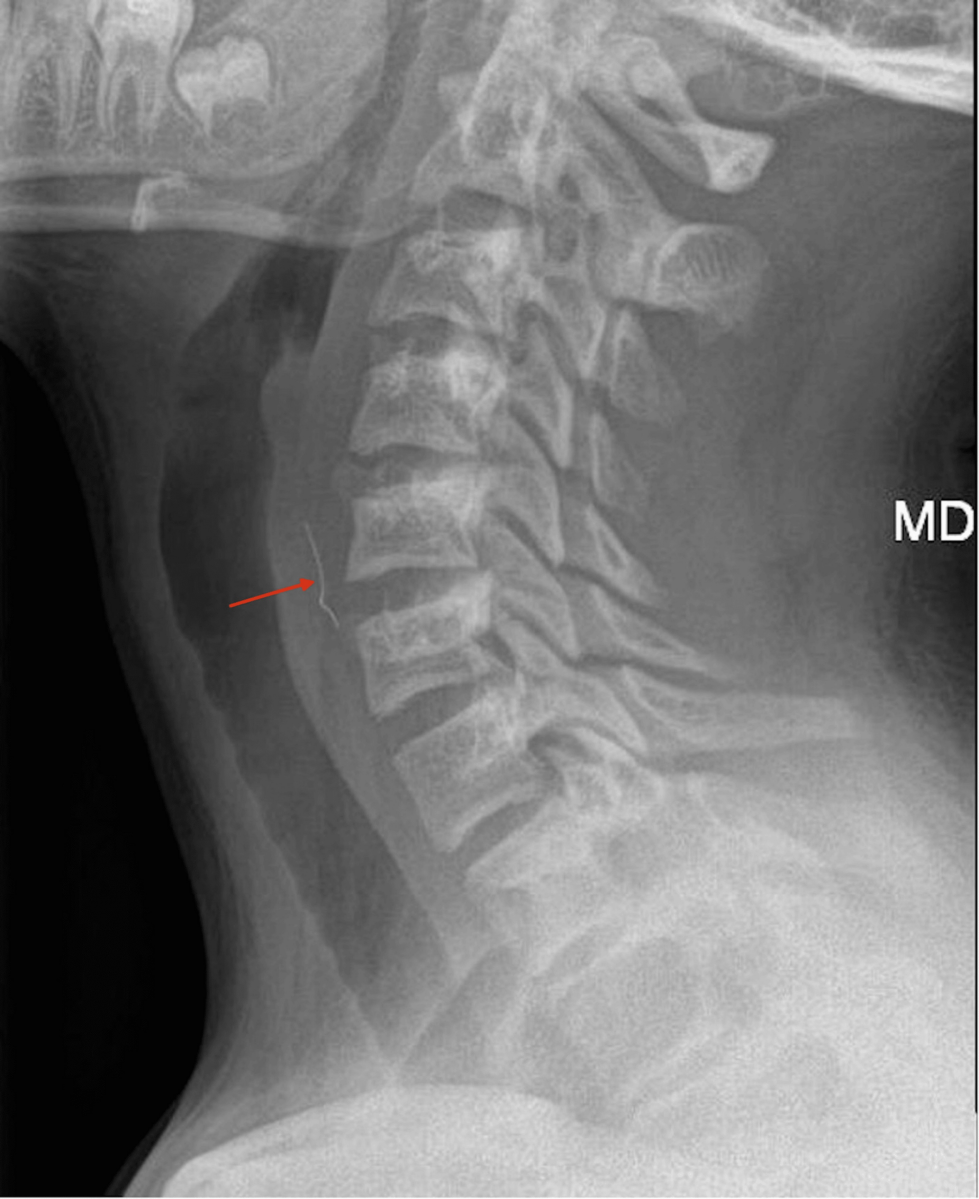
Lateral soft tissue neck X-ray demonstrating a linear opacity projected over the esophagus at the C5-C6 vertebrae level (red arrow).
